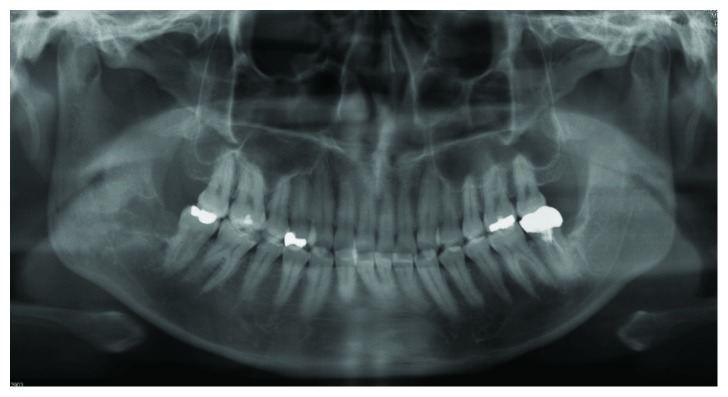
A panoramic radiograph obtained during the initial visit. The radiograph shows radiolucency from the extraction socket to the right mandibular angle.
